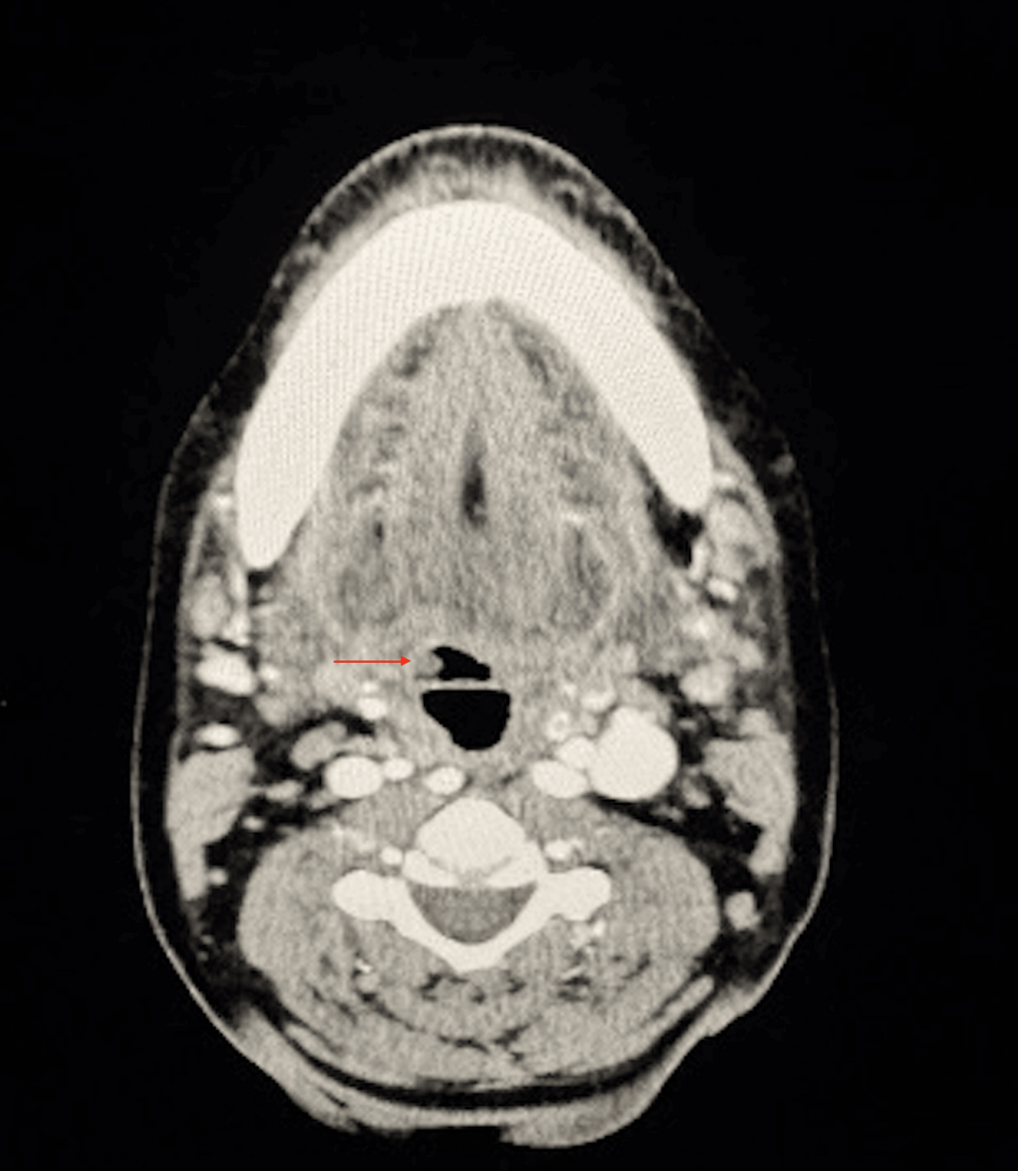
Axial CT image showing a small lesion arising from the right vallecula (red arrow).
